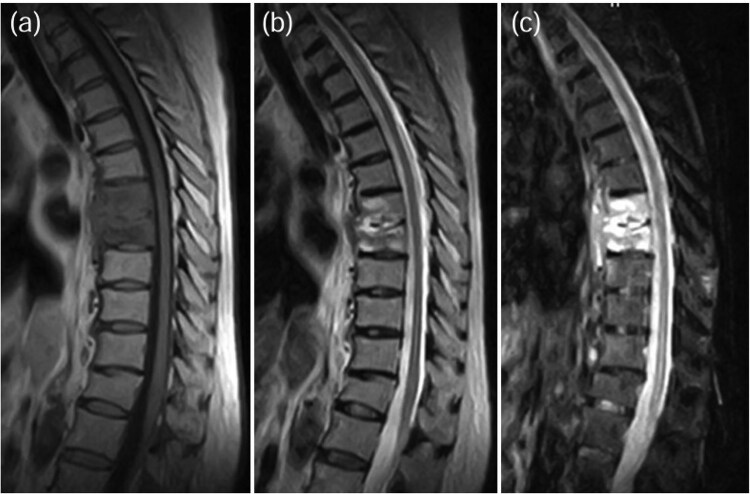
MRI demonstrated signal changes at Th7-Th8, with low intensity on T1-weighted images (a), high intensity on T2-weighted images (b), and no suppression on short-tau inversion recovery (STIR) images (c).
